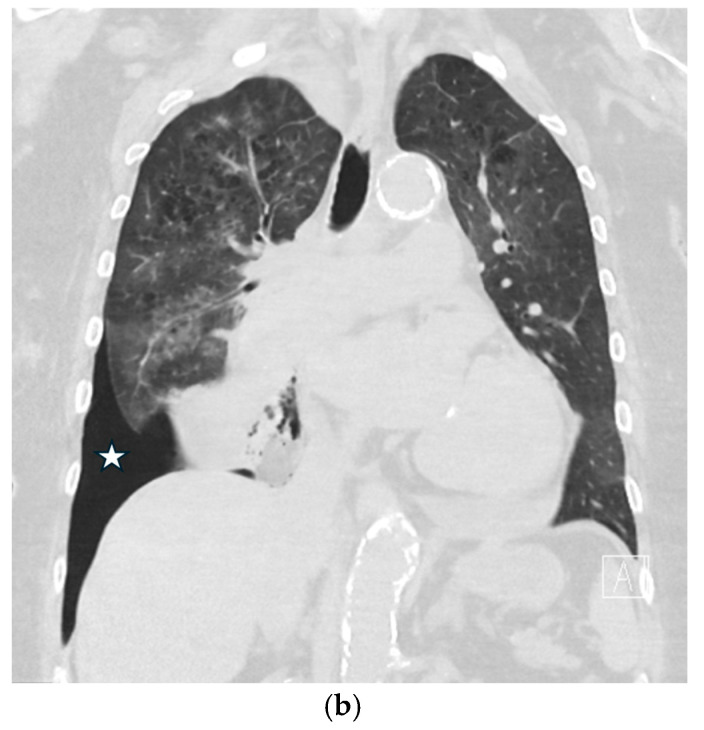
74-year-old female patient status post-cardiac arrest at home. She was resuscitated by the emergency medical services at home and arrived at the emergency department with a Glasgow coma scale of 3. The initial portable chest radiograph (a) demonstrated a right-sided deep sulcus sign (white arrow). Coronal image (b) from CT performed on the same day demonstrates a large right basilar pneumothorax (star).
